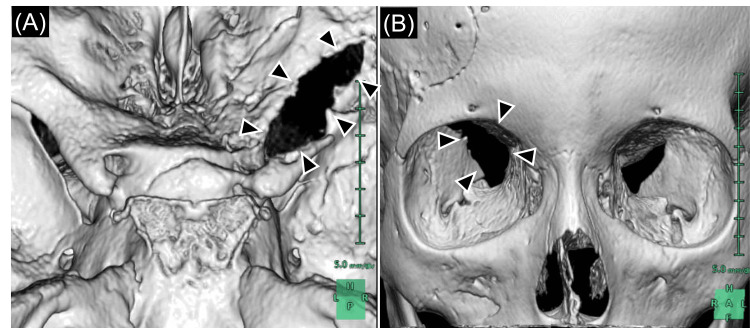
Three-dimensional computed tomography (3D CT) taken following optic canal decompression (A, B): 3D CT revealing the decompression of the right optic canal postoperatively (black arrowheads).
